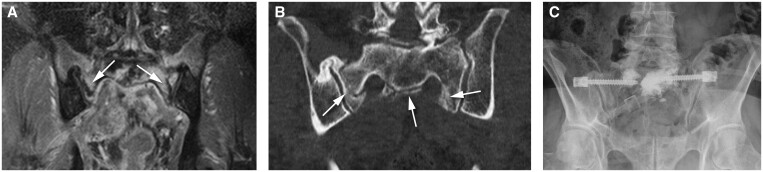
Bilateral sacral IF visible (*arrows*) on T1W SPIR Gd-enhanced MRI (A) and on CT scan (B) with a *“H”*-shape, with a vertical component parallel to the articular surface of the SI joint and a continuous transversal component that crosses the sacrum at the level of S1, prior to the stabilization surgery; (C) radiograph following the stabilization surgery using cementoplasty with placement of screws crossing the SI joint as well. CT = computed tomography; IF = insufficiency fractures; MRI = magnetic resonance imaging; SI = sacroiliac.
